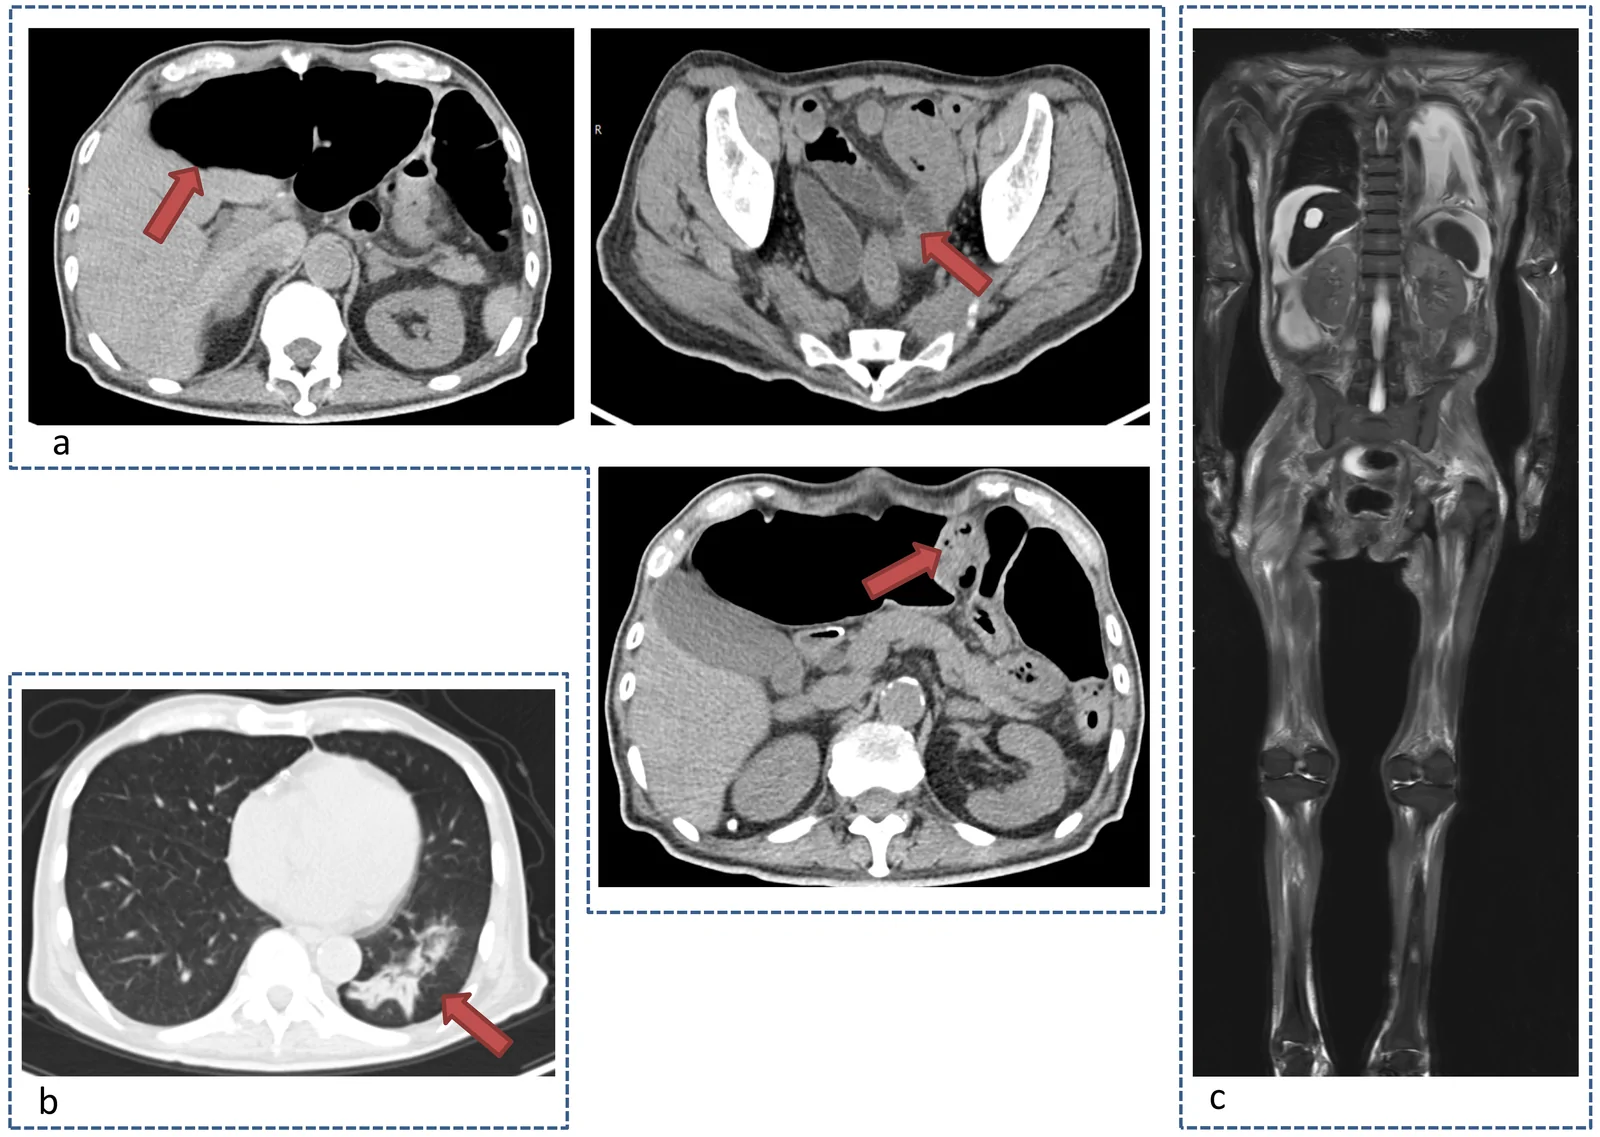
PET/CT and MRI findings showing intestinal pseudo-obstruction and multisystem inflammatory involvement. (a) PET/CT showed diffuse gaseous distension of the bowel loops, without a definite site of mechanical obstruction, mass lesion, or fixed transition point. Postoperative changes related to gastrojejunostomy were noted. (b) PET/CT showed patchy inflammatory exudative opacities in the left lung. (c) Whole-body muscle MRI showed multifocal hyperintense signals in the trunk, pelvic, and lower-limb muscles, accompanied by adjacent soft-tissue edema.
